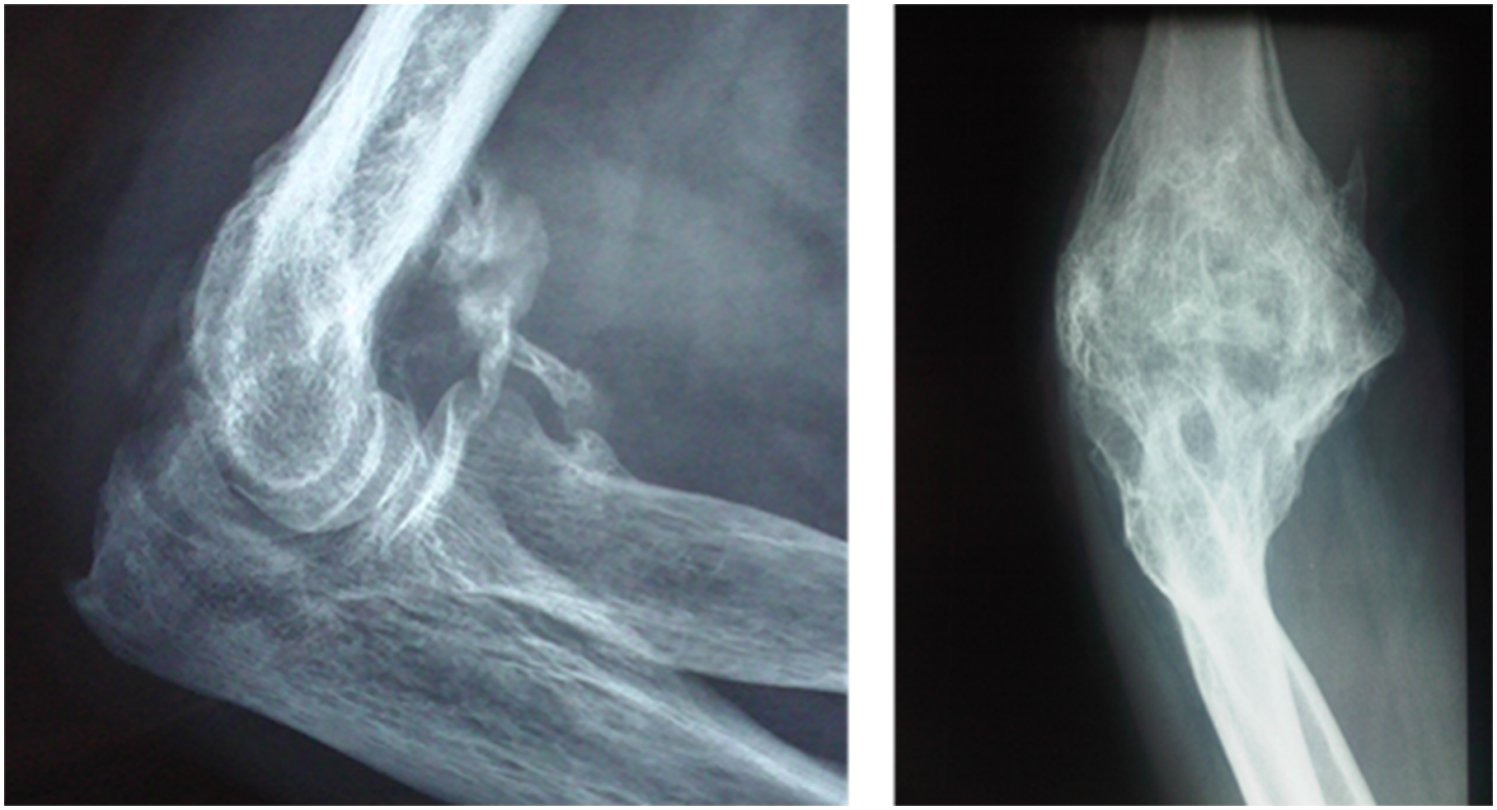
Heterotopic ossification around the elbow in patients with burns. A and B: X-ray images of circumferential HO.
